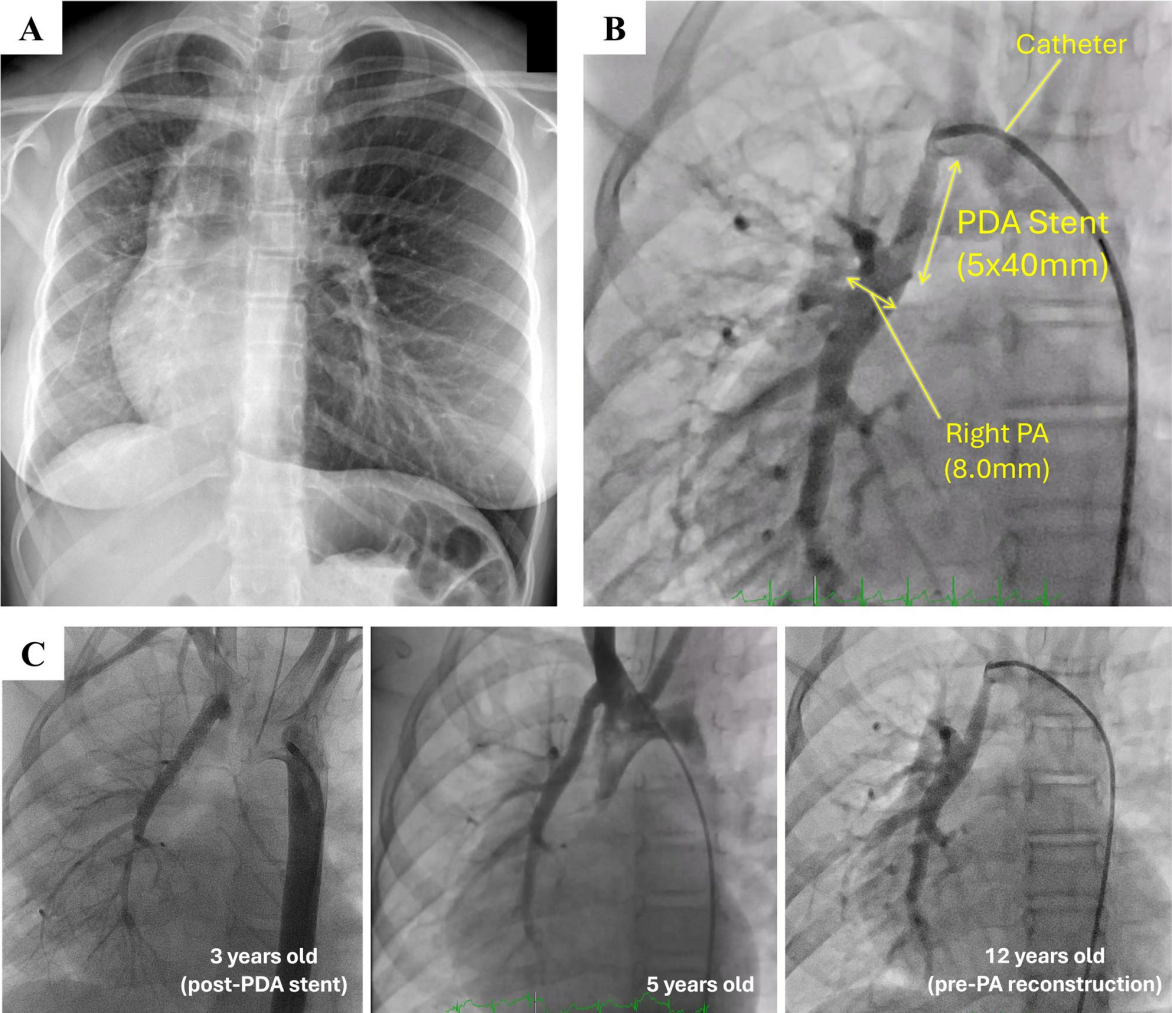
A: Preoperative Chest X-ray, B: preoperative cardiac catheterization, C: catheterization series
